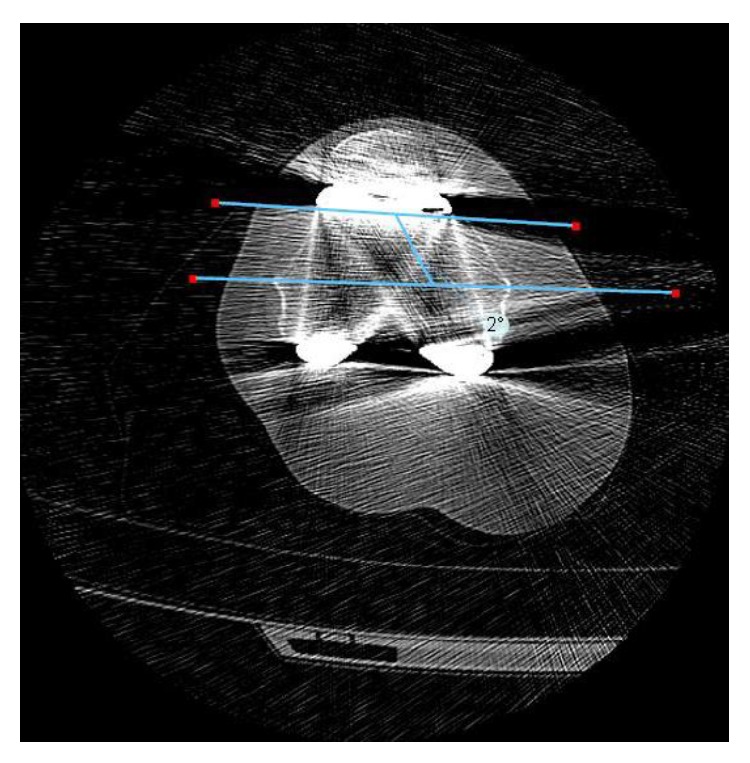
CT-scan on operative knee (after surgery). Measurement of femoral rotation in regard to anatomical epicondylar axis performed by two independent observers.
